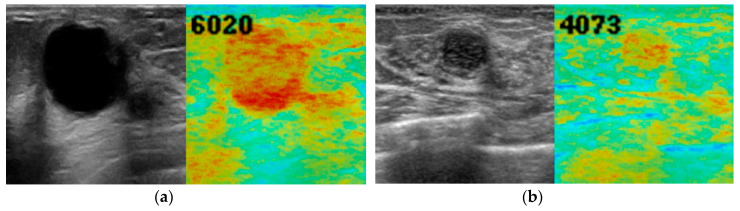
B-mode ultrasound images (left, grey) and anomaly score images (right, color) of breast with benign masses. A 46-year-old woman with intraductal papilloma (a) and a 58-year-old woman with fibroadenoma (b). There was abnormal red color consistent with the masses. The anomaly score was moderate medium to high (6020 and 4073, respectively).
